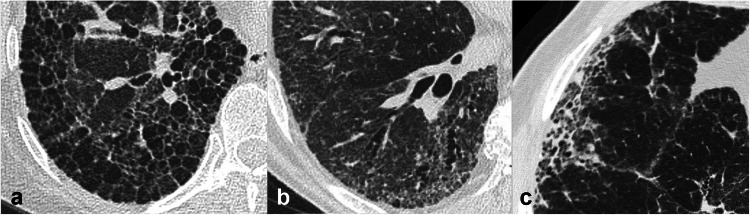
Computed tomography (CT) signs of pulmonary fibrosis. Honeycombing, characterised by destruction of lung parenchyma replaced by well-defined cystic spaces with thick walls, typically clustered in multiple layers in the subpleural region (a). Traction bronchiectasis, characterised by bronchial irregular dilatation in a background of intralobular fibrosis. Note the mild volume reduction of the right lower lobe, demonstrated by the posterior displacement of the major fissure (b). Fibrotic reticulation with irregular thickening of the interlobular and intralobular septa, associated with distal traction bronchiolectasis, in the subpleural region (c). Architectural distortion of the lung is present in all CT images
